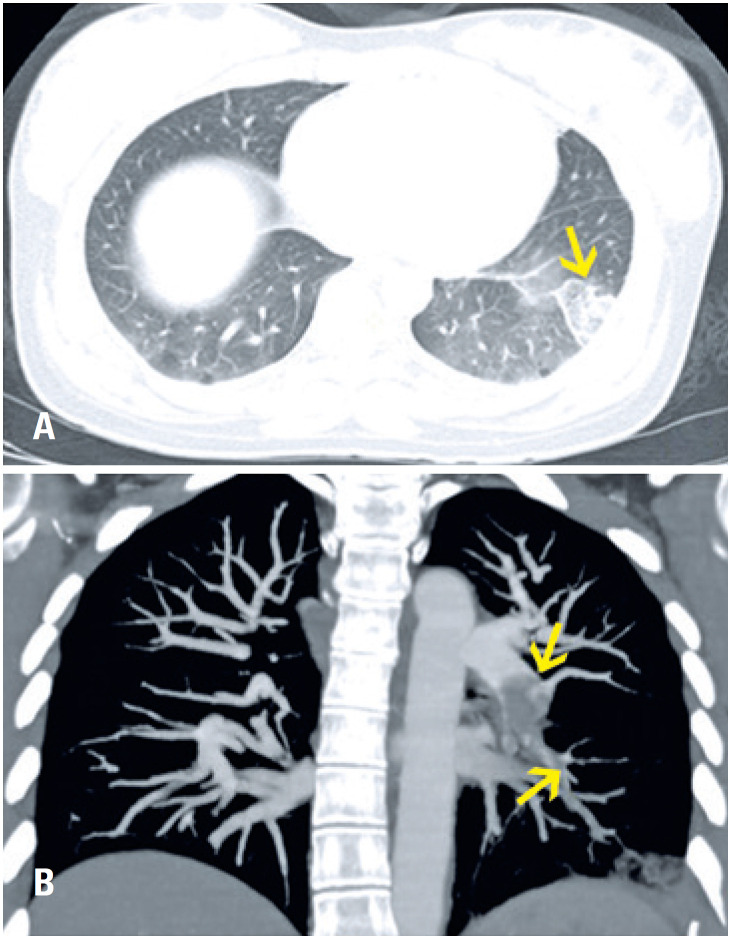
A 30-year-old woman complaining of sudden dyspnea. Computed tomography (A) Axial section and (B) Coronal reformation image with reconstruction (maximum intensity projection) of the soft tissue window: positive computed tomography angiography for pulmonary thromboembolism, with filling defects in the left interlobar artery and the segmental branches for the left lower lobe. Small reversed halo opacity. The opacity is wedge-shaped, with the apex facing the subpleural hilum in the lateral basal segment of the left lower lobe, which may correspond to the area of pulmonary infarction
